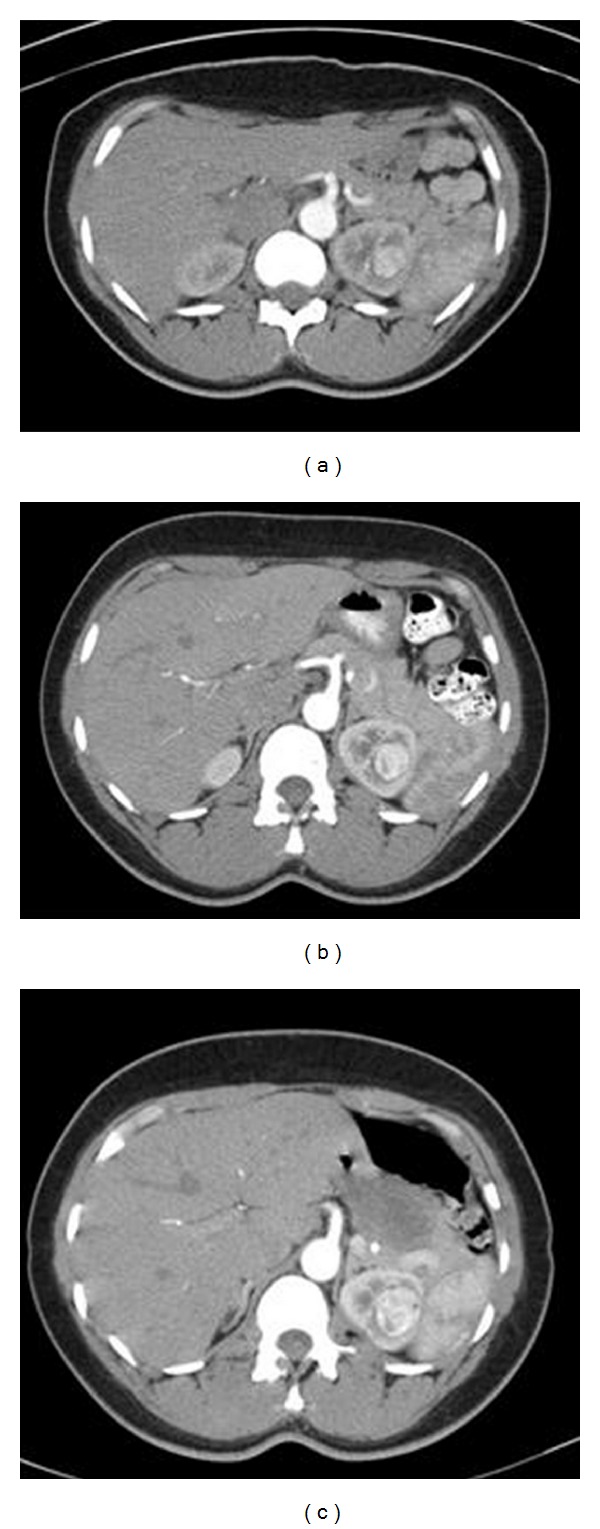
Serial contrasted CT scan of the abdomen demonstrating interval growth of enhancing left renal mass (12, 8, 4 months prior to partial nephrectomy).
